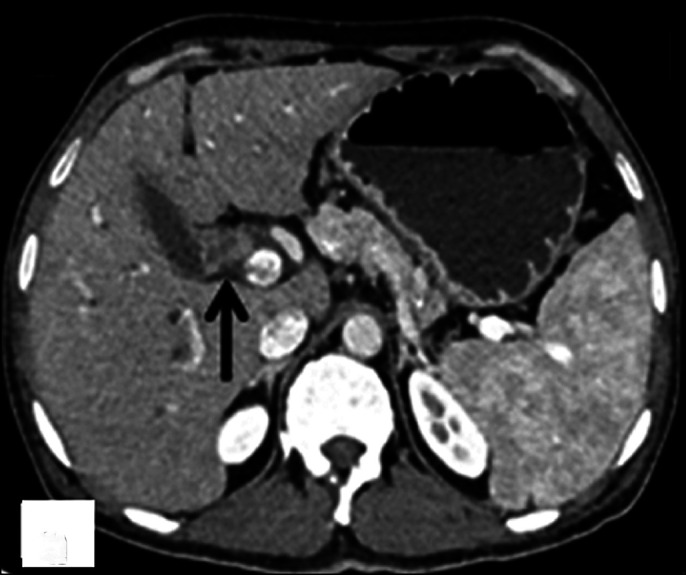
Abdominal contrast-enhanced computed tomography showing thickening at the gallbladder neck with extension along cystic duct and proximal common bile duct (arrow).
